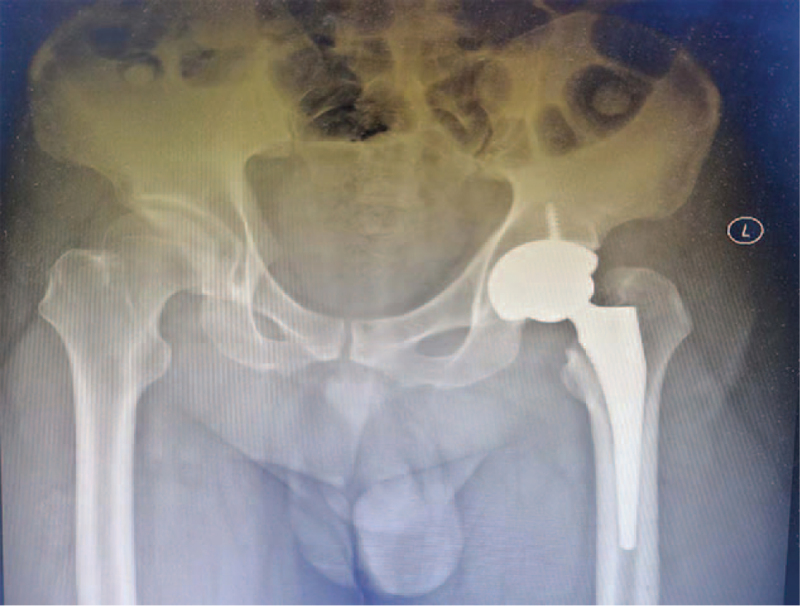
Pelvic plain radiograph showing postleft total hip arthroplasty.
